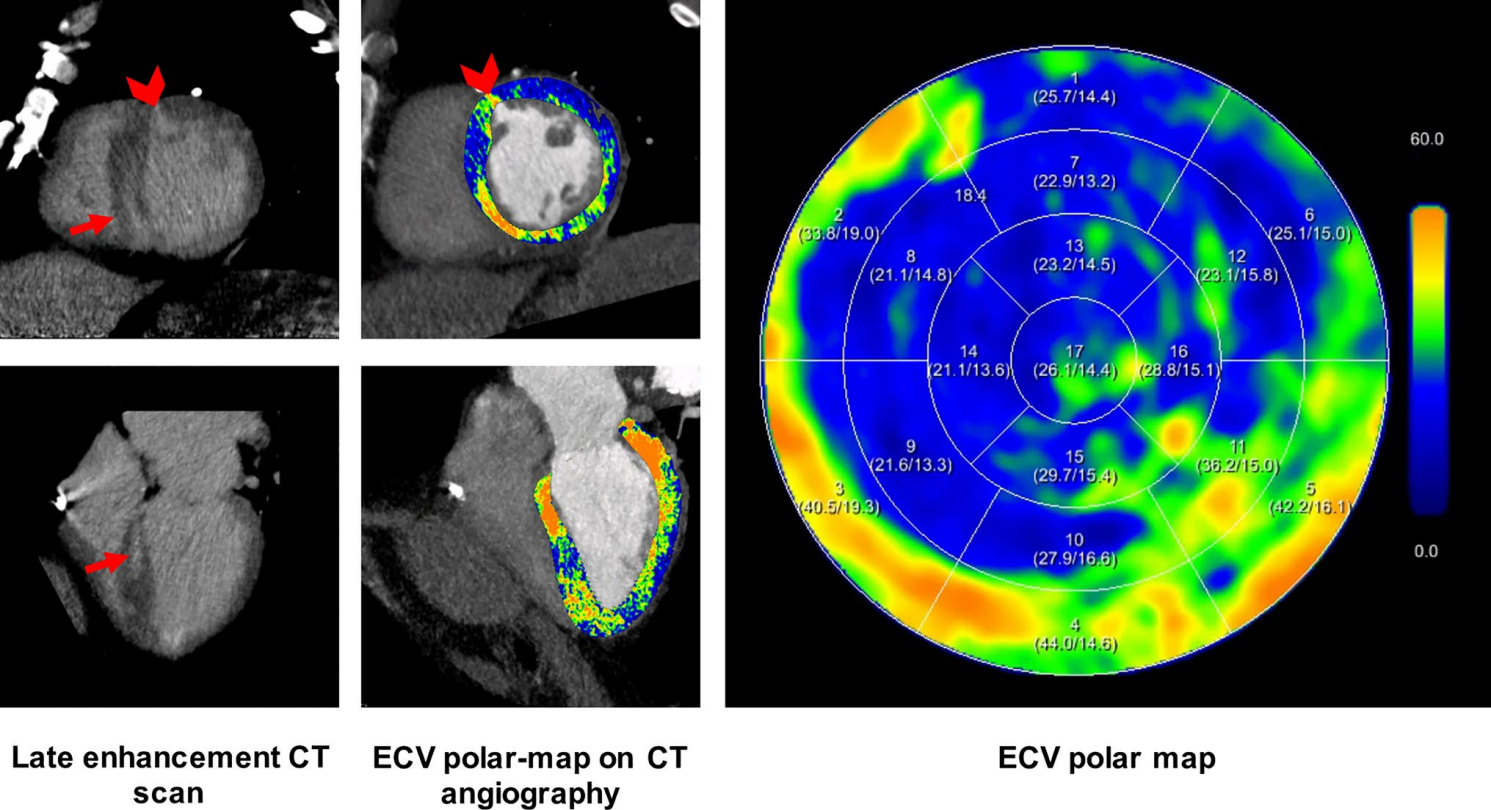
Eighty two-year-old female patient with severe aortic stenosis and known chronic ischemic cardiomyopathy. Late iodine enhancement images and iodine maps document increased myocardial late enhancement in the basal infero-septal left ventricular wall (red arrow in late enhancement CT). Elevated ECV values (patient hematocrit 43%) are shown in the corresponding territory. In addition, a tiny area of myocardial late enhancement is seen at the basal antero-septal junction (arrowhead in short axis late enhancement CT and ECV polar map), which may be related to aortic stenosis. Elevated ECV values are also shown in the basal infero-lateral segment, as the result of a known previous myocardial infarction
